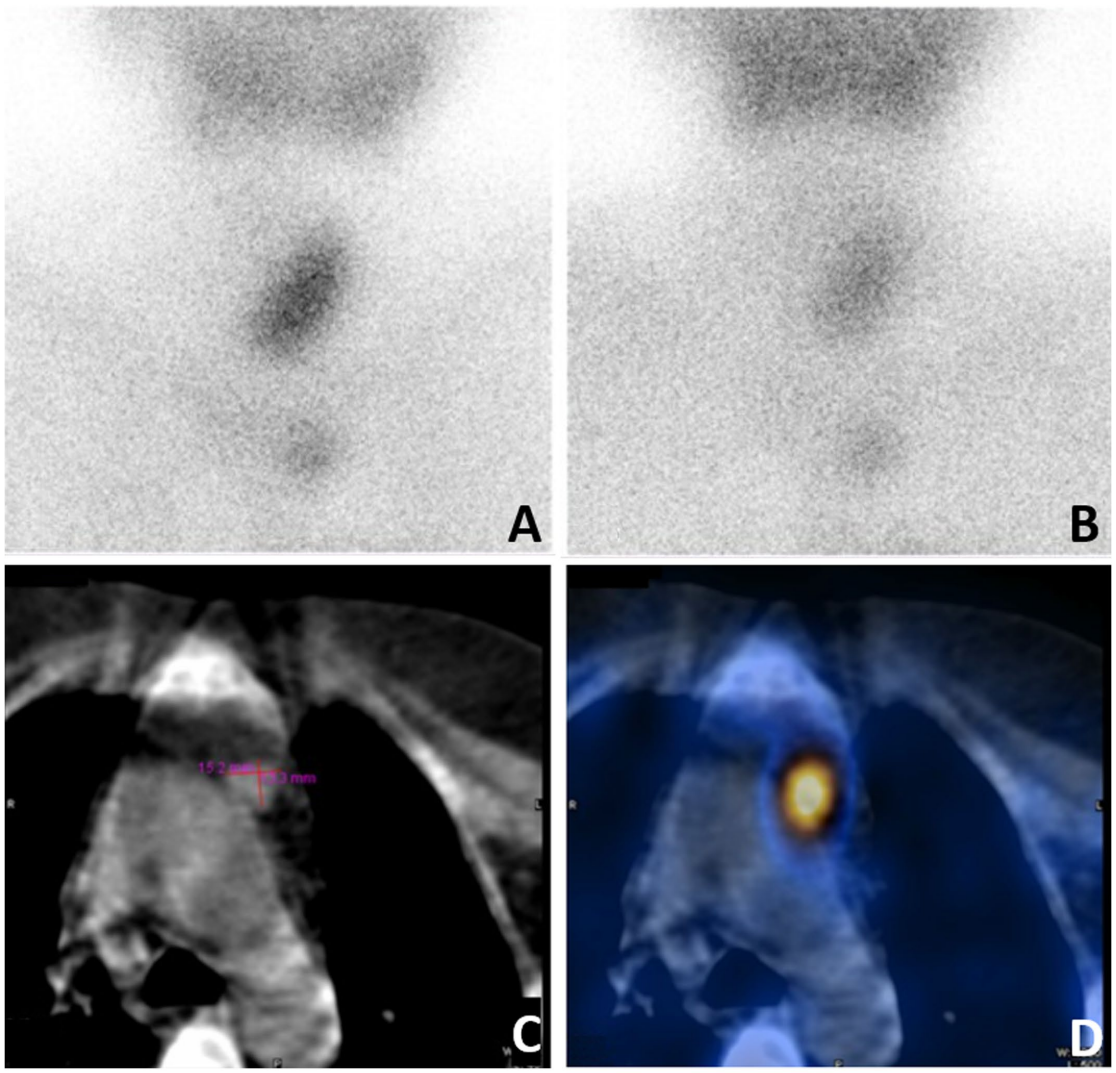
Reexamination of ^99m^Tc-MIBI and SPECT/CT before the second operation. (A)
